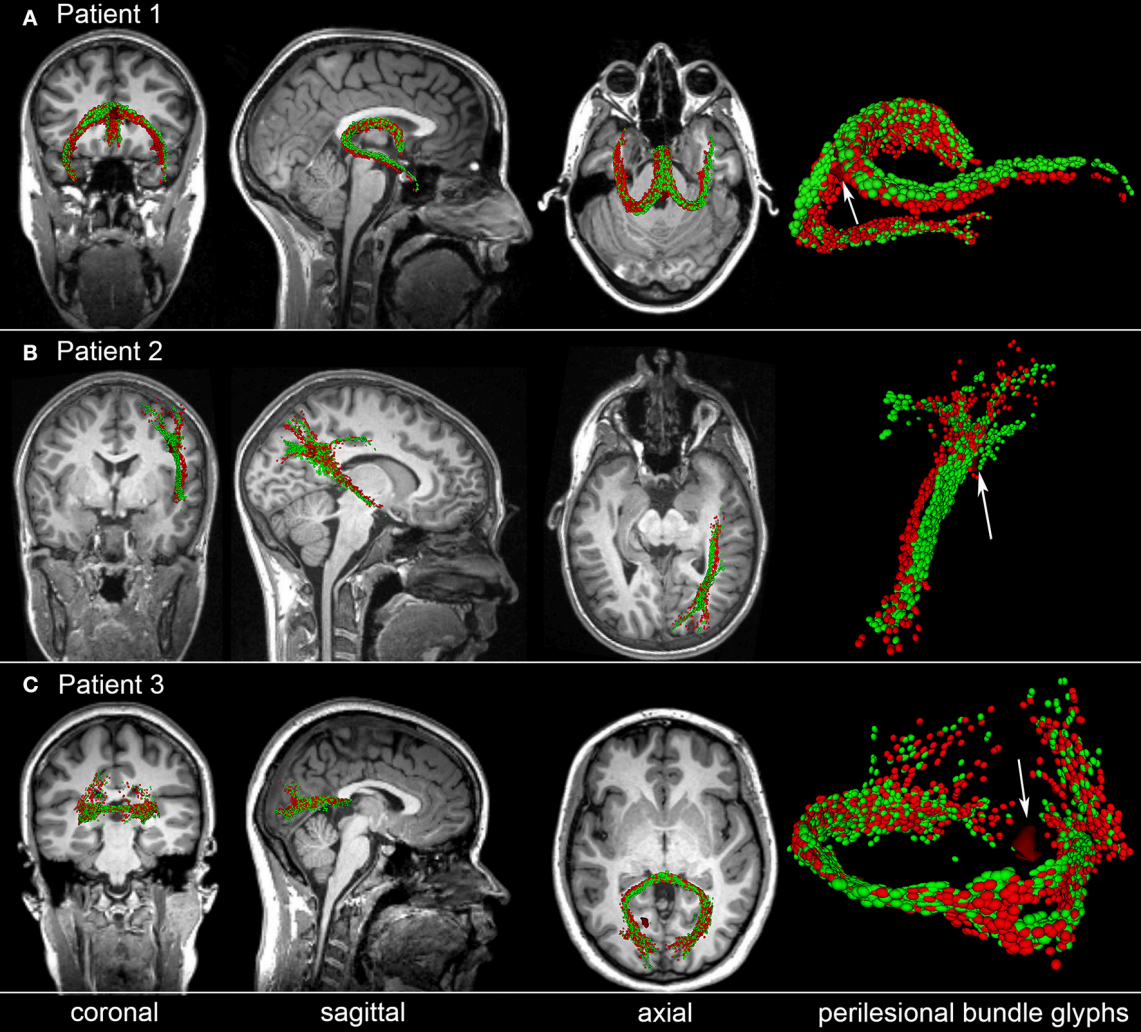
Representative examples of perilesional streamlines in three TBI victims. For each case, standard views (coronal, sagittal, axial) of *T*_1_-weighted MRI are shown in addition to DTI glyphs associated with perilesional WM streamline bundles imaged acutely (red) and chronically (green). White arrows indicate CMB locations. (A) Patient 1 exhibits a CMB near the fornix, near the hippocampal commissure. Six months after injury, the location and trajectory of the right fimbria are visibly different from their acute presentation. (B) Patient 2 has a CMB in the deep WM, near connections between the right parietal and temporal lobes; glyph comparison indicates a spatial shift of the longest streamline bundle toward the longitudinal fissure. (C) Patient 3 has a hemorrhage close to a left-hemisphere streamline bundle belonging to the splenium of the corpus callosum. The splenium is notably asymmetric at both time points, with the asymmetry being close to the CMB. If a causal relationship between the CMB and this neuroanatomic correlate could be established, this example would provide proof-of-concept that some CMB-related alterations in brain structure can persist for months after the traumatic event.
